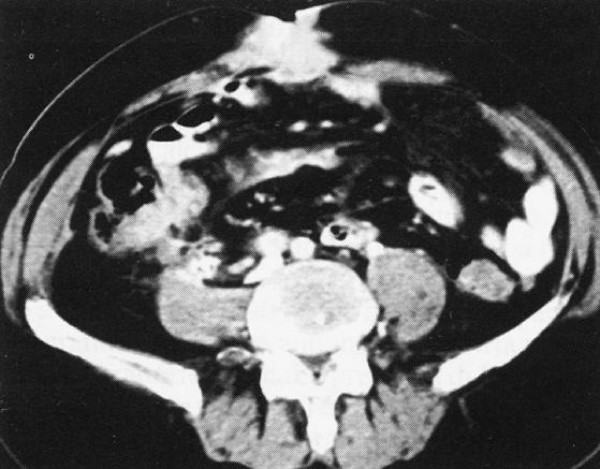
CT-scan of the abdomen: This image shows an umbilical mass measured 3 cm, with indefinite margins.
